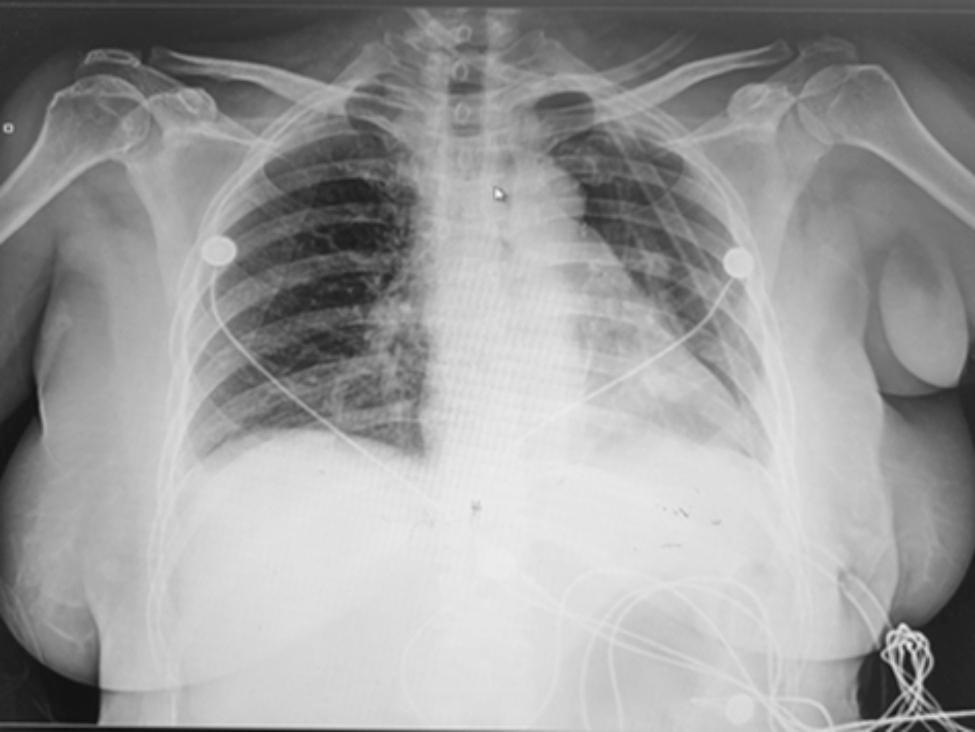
Postoperative chest X-ray showed good recruitment of the left lung without obvious pleural effusion
